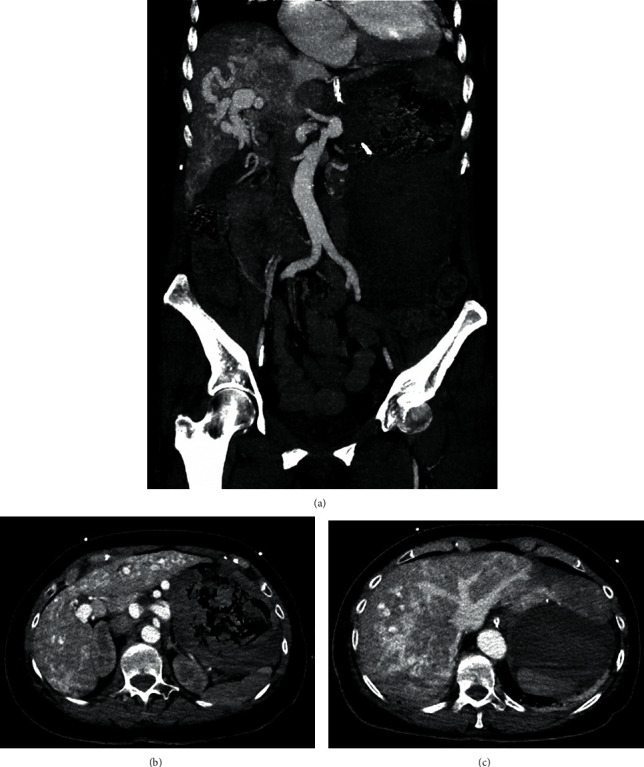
Coronal and axial late arterial phase abdominal CT imaging demonstrating hepatic arteriovenous shunting. (a) Coronal reconstruction showing hepatic arterial enhancement, the portal venous system is not opacified with contrast. There is early contrast filling of the hepatic veins and the inferior vena cava consistent with the hepatic arteriovenous shunting. (b) Axial slice through the level of the portal vein shows no contrast opacification of the portal system. (c) Axial slice through the level of the hepatic vein shows contrast enhancement. Incidentally noted is a markedly dilated stomach filled with blood products from gastrointestinal angiodysplasia.
